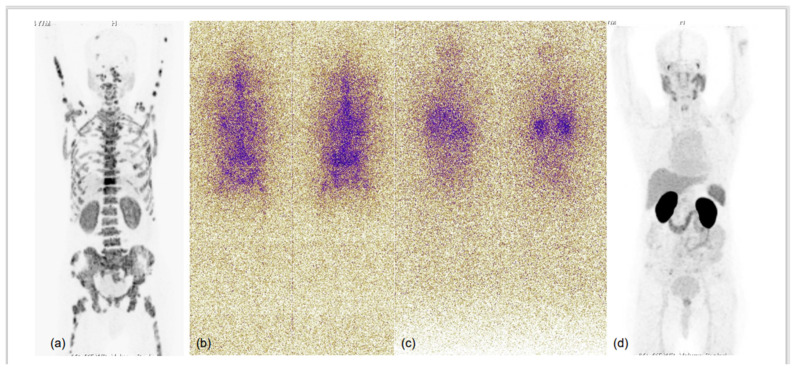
75-year-old male with known case of coronary artery disease, post-CABG and on cardiac remodeling agents, presented with multiple sites of skeletal pain, weakness and high serum PSA >500.0 ng/mL. Needle biopsy from prostate demonstrated acinar adenocarcinoma (Gleason score 4 + 3 = 7), underwent bilateral orchidectomy. ^68^Ga-PSMA-11 PET-CT performed for high-serum PSA showed low-grade PSMA expression in relatively smaller prostate gland, with no significant PSMA-expressing or otherwise pelvic and retroperitoneal lymph nodes and PSMA-expressing sclerotic and marrow metastatic lesions involving entire visualised skeleton. In view of cardiac co-morbidity and post-CABG status, chemotherapy and anti-androgen therapies were not considered, and he was taken for ^225^Ac-PSMA-617 (alpha radionuclide) therapy. MIP images of ^68^Ga-PSMA-11 PET-CT scans at baseline (a) and 3 months after second cycle of ^225^Ac-PSMA-617 (d), and first (b) and second (c) post alpha therapy planar gamma scans showed excellent scan response; PSA decreased to 0.405 ng/mL and patient became asymptomatic.
